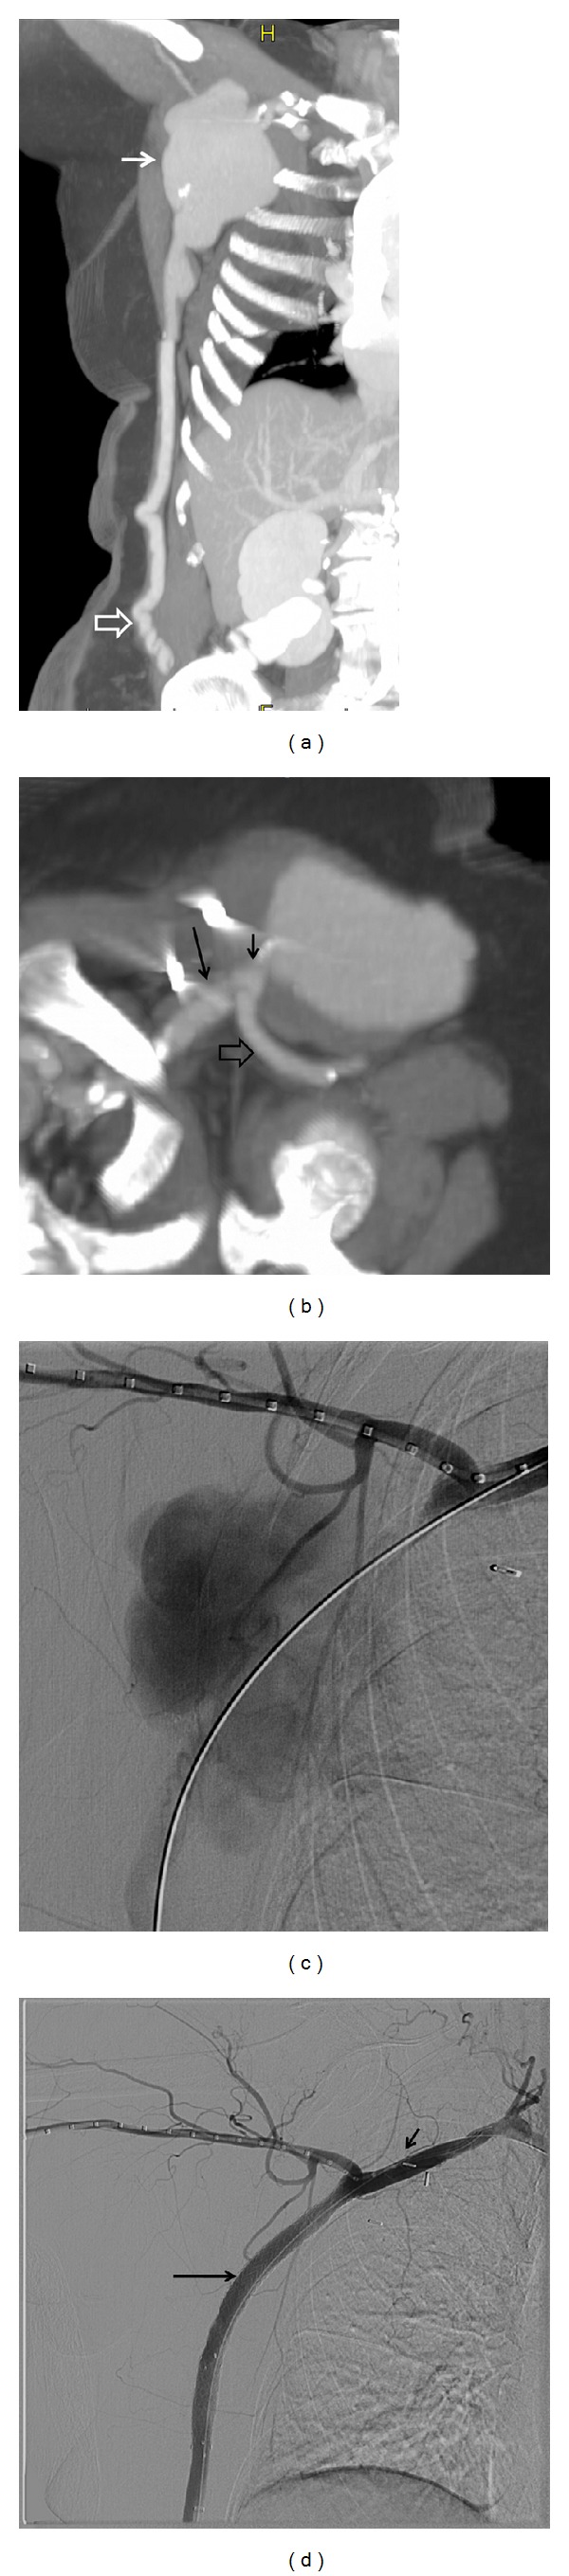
(a) Coronal CT scan demonstrates a large aPSA near the proximal end of an axillofemoral bypass graft (arrows). The distal end of the bypass is noted by the open arrow. (b) A reconstructed para-axial image from the CT scan better shows the relationship between the aPSA and proximal bypass anastomosis. Long arrow corresponds with the axillary artery with the open arrow pointed at the axillary vein. A short landing zone for stent graft exclusion is noted at the neck of the aPSA (short arrow). (c) Angiogram shows filling of a large aPSA located 2 cm from the subclavian artery anastomosis. A marker catheter has been introduced from the brachial access and a wire traverses the graft and extends into the proximal subclavian artery via a direct distal bypass puncture. (d) Successful complete exclusion of PSA is seen on completion arteriography with preserved flow through both the native subclavian (short arrow) and brachial artery as well as the axillobifemoral graft (long arrow).
